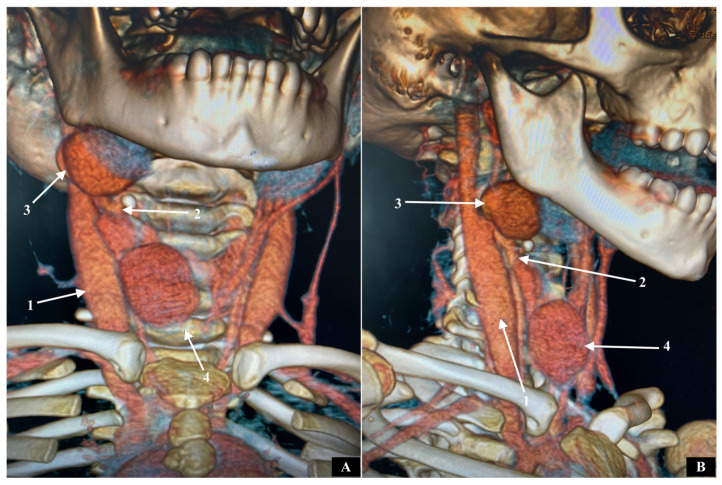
(A) Anterior view. (B) Right lateral view. CTA shows Two aneurysms of a right communicating vein. 1: right internal jugular vein; 2: right communicating vein; 3: aneurysm of the right communicating vein in the retro-parotid area; 4: aneurysm of the right communicating vein above the jugular notch.
